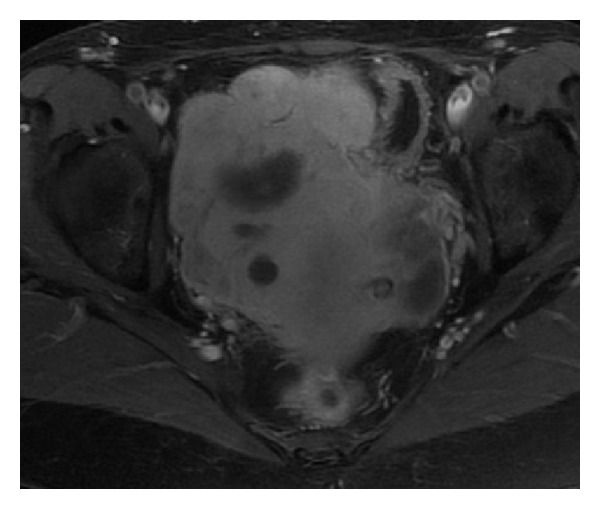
Lobulated mass, filling the pelvis and indenting uterus in MRI.
